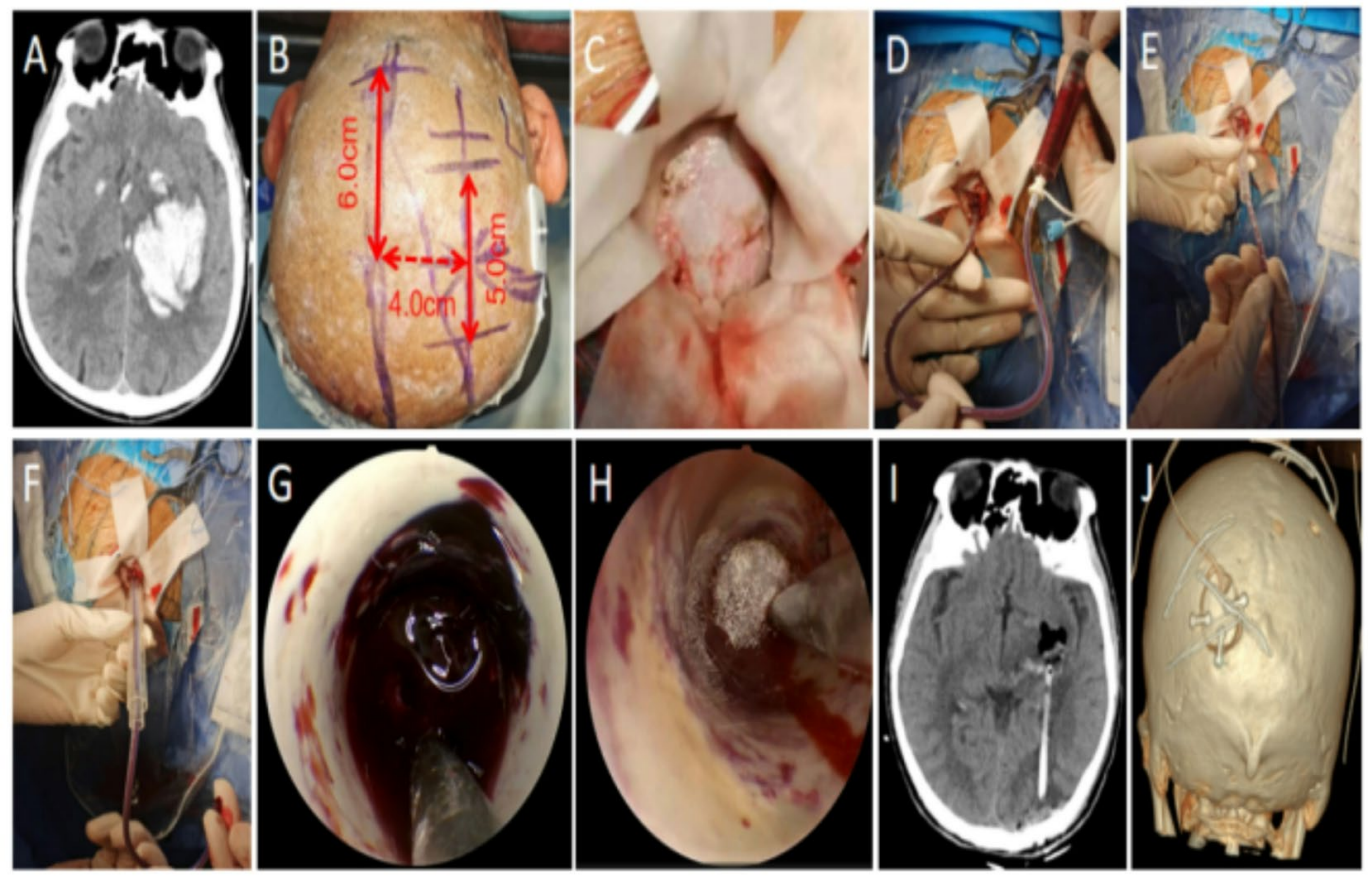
Surgical procedure for left posterolateral thalamic hemorrhage. (A) Head CT showing left posterolateral thalamic hemorrhage. (B) Surgical path planning based on head CT and skin mark. (Skin marker location was decided based on preoperative CT reconstruction.) (C) Surgical incision 5 cm and bone window 2.5 cm x 3.0 cm. (D-F) Guide-wire drainage tube aspiration confirming position within hematoma cavity; 10–20 ml aspirated; sequential insertion of primary and secondary dilator sleeves; removal of drainage tube and primary sleeve. (G-H) Endoscopic hematoma evacuation; hemostatic material covering cavity post-evacuation. (I-J) Postoperative head CT showing satisfactory evacuation of left thalamic hematoma; 3D reconstruction showing left occipital keyhole approach.
